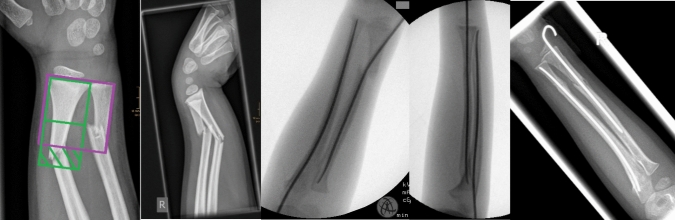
a–e. a, b a.p. and lateral radiograph of a diametaphyseal forearm fracture in a 9-year-old boy. The fracture lies within the diametaphyseal junction zone (DMJZ) as defined by a forearm fracture index between 1 and 2, but proximal to the metaphysis as defined by the AO-PCCF. This fracture can hence be categorized as DMRF+. c, d intraoperative a.p. and lateral radiographs after reduction and osteosynthesis with elastic stable intramedullary nailing (ESIN). The distal radial fragment presents with translation and ulnar deviation in the a.p. radiograph. e a.p. radiograph after removal of the ESIN osteosynthesis and reduction and transepiphyseal percutaneous intramedullary Kirschner-wire fixation (TEPIK) of the fracture. Angulation and translation of the distal radial fragment have disappeared
